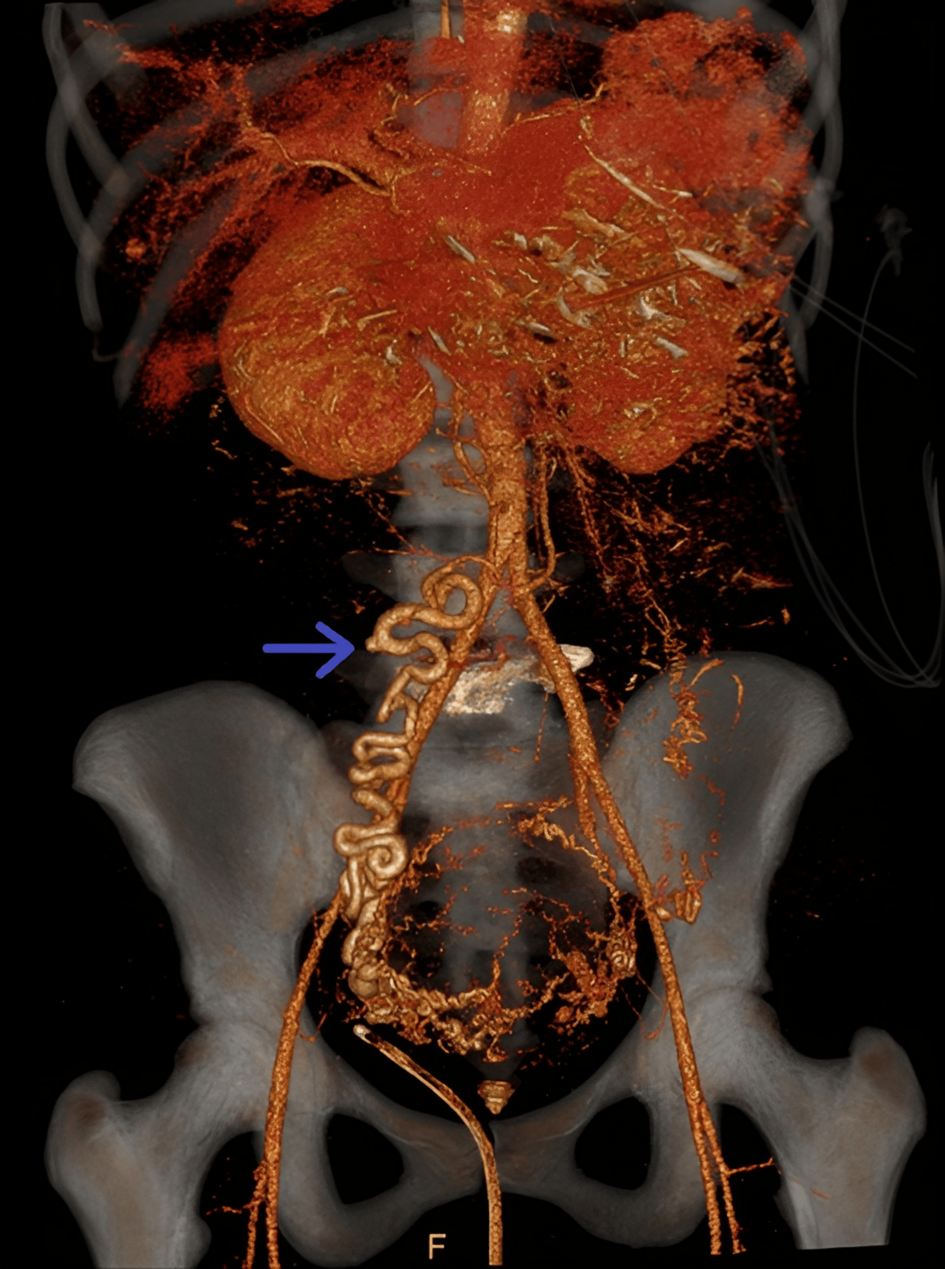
Coronal view of a three-dimensional reconstruction of a CT angiogram scan of the abdomen, with the blue arrow demonstrating the aberrant artery.
